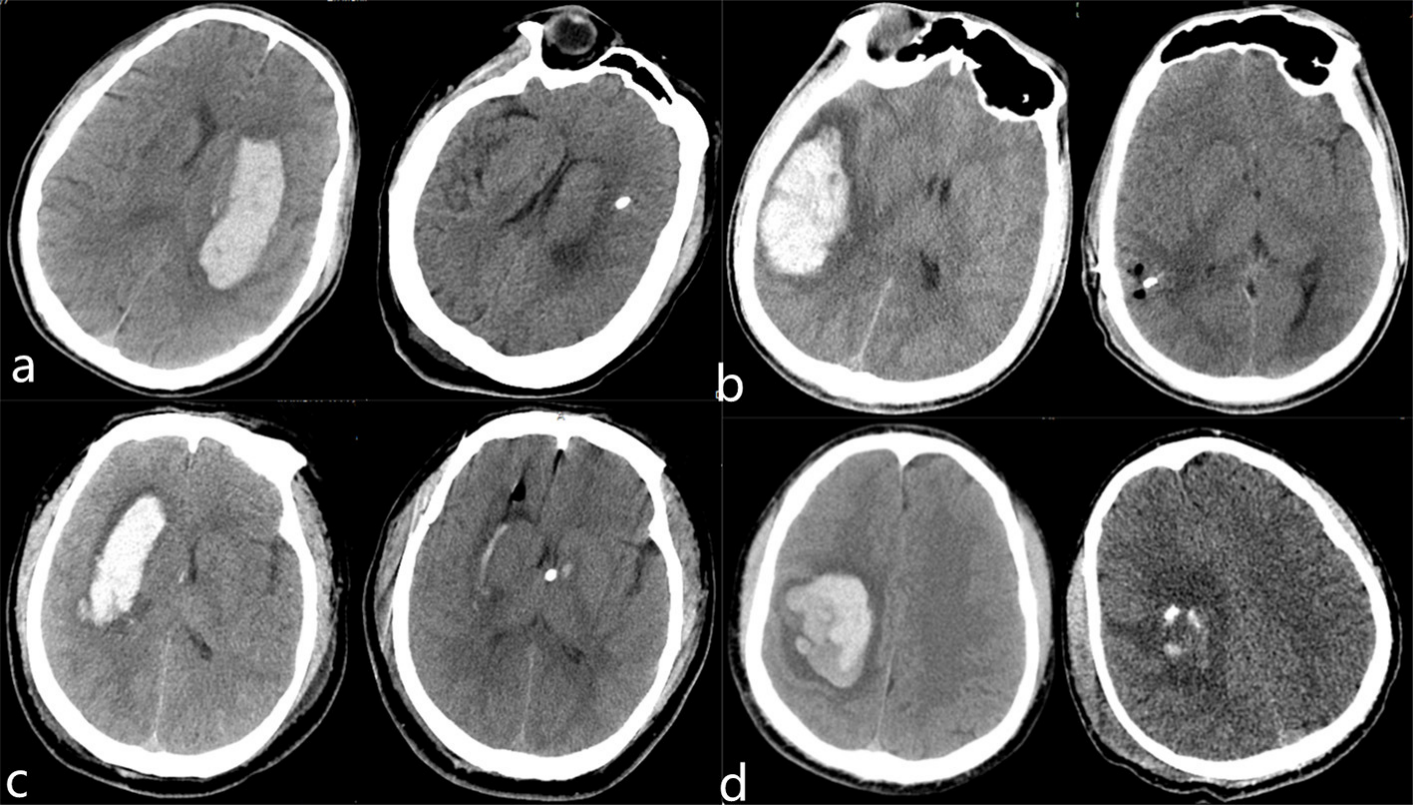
Preoperative and postoperative CT images in robot-assisted neuroendoscopic hematoma evacuation combined ICP monitoring of representative patients with HICH (a–d). (a) A 62-year-old female was presented with a headache and right hemiplegia for 4 h. The hematoma was removed completely and the symptoms of headache were improved obviously. Continuous rehabilitation training was given to the patient after discharge. (b) A 54-year-old male presented with a headache for 10 h. Preoperative CT shows right temporal lobe hemorrhage that has been evacuated totally. (c) A 65-year-old male was presented with consciousness disturbance for 5 h. Preoperative CT scan shows right basal ganglia hemorrhage, which has been evacuated and we inserted a drainage tube with the Codman ICP monitoring probe to the ventricle. (d) A 73-year-old male was presented with left hemiplegia for 3 h. Preoperative CT shows right parietal lobe hemorrhage, which has been evacuated and we inserted a drainage tube with the Codman ICP monitoring probe to the hematoma cavity. Continuous rehabilitation training was given to the patient after they were discharged.
